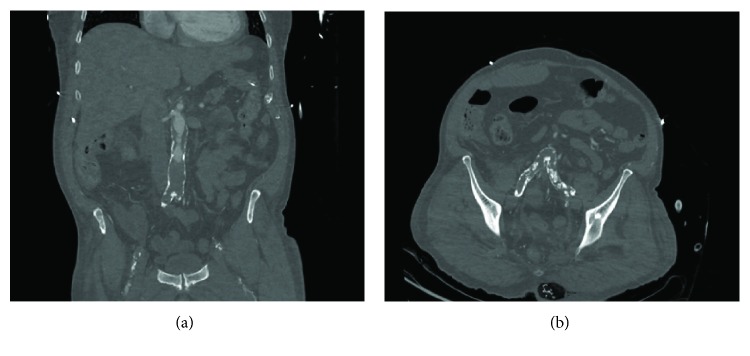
The CT angiography scan revealed extensive aortoiliac occlusion. (a) Occlusion of the distal aorta. (b) The thrombus extends into the iliac arteries.
